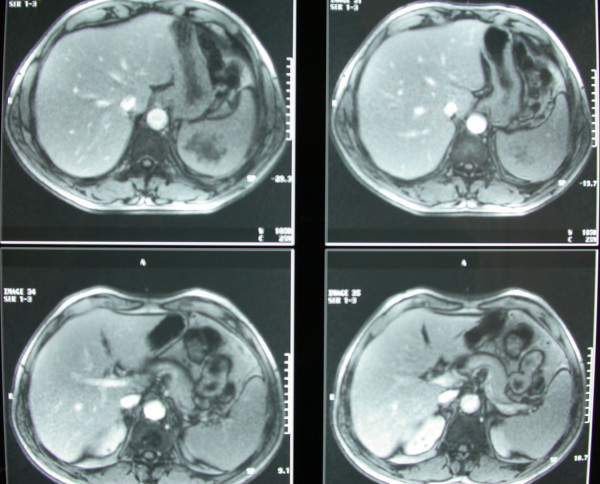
MRI of the upper abdomen showing a massive solitary lesion in the spleen with a low-density core and a peripheral contrast enhancement suggestive of metastasis.
